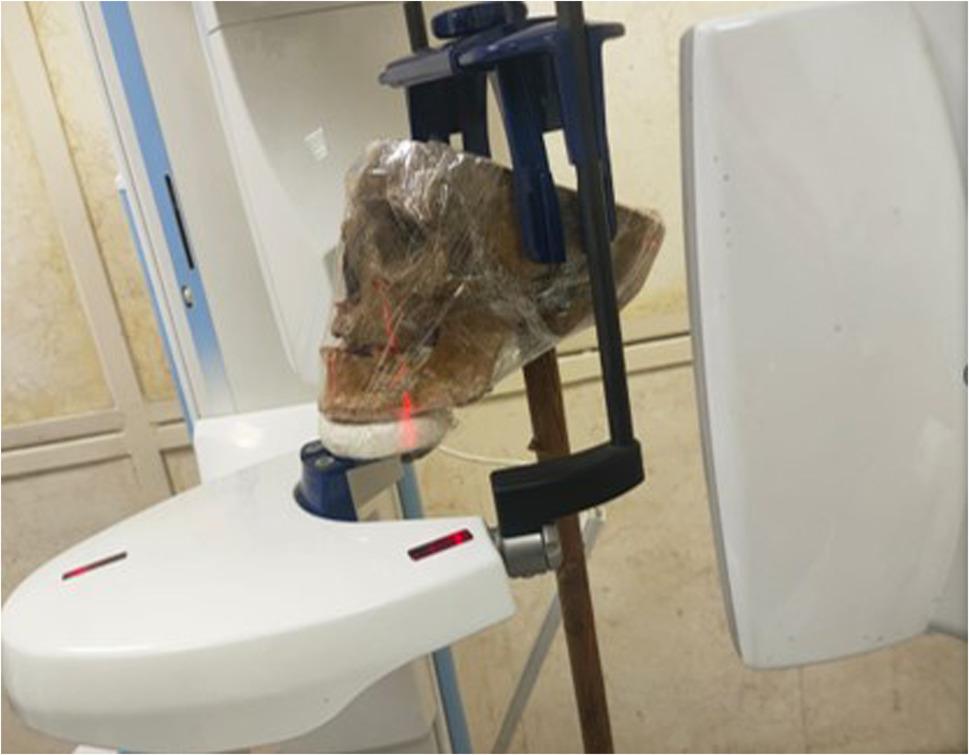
A photograph showing the position of the assembled skull on the CBCT machine
